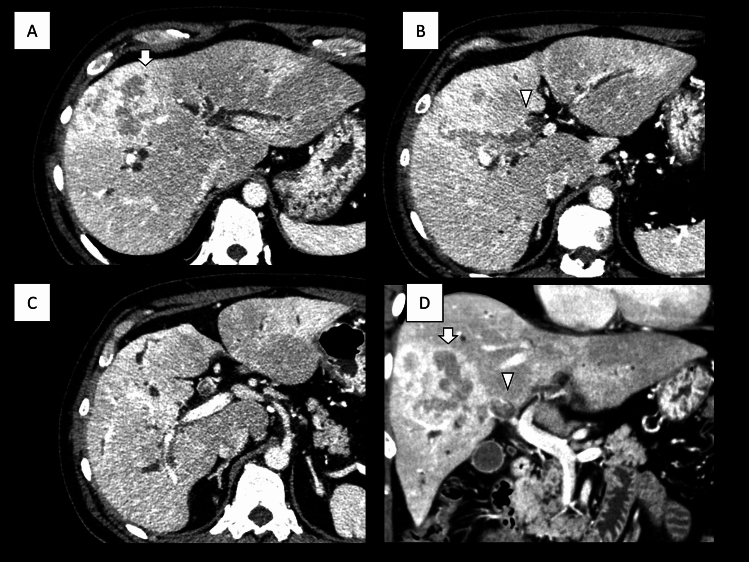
Abdominal computed tomography showing a 10 cm tumor in the right liver (white arrow), tumor invasion into the hilar bile duct, tumor thrombi in the common bile duct (white arrowhead), and dilated right and left intrahepatic bile ducts; A, B, and C are axial cross-sections; D is the coronal cross-section
